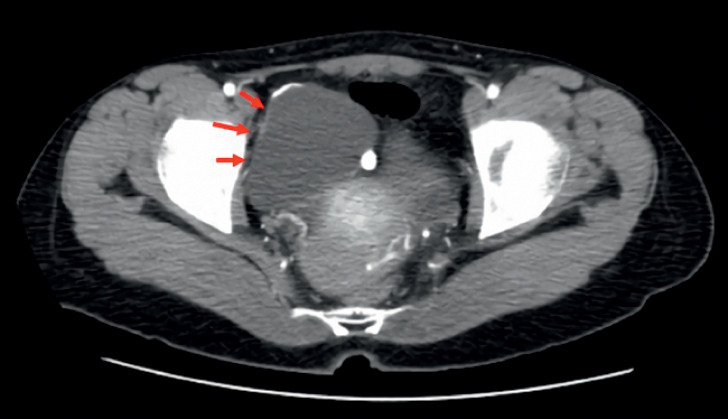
Abdominal enhanced computed tomography (CT), suggesting an irregular cystic low-density shadow on the right side of the pelvis.
